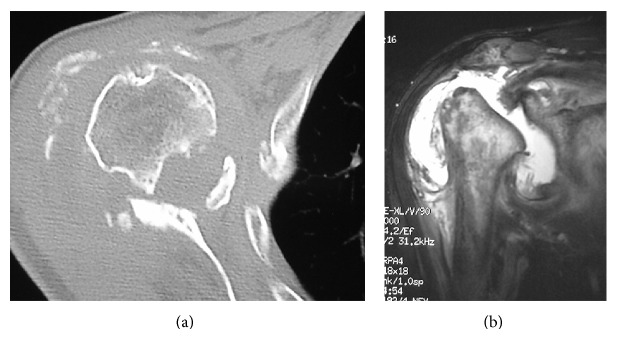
Case  1. Imaging of the right shoulder 4 months after the first visit. (a) An axial computed tomography view demonstrates a destroyed humeral head, displaced bony fragments outside of the greater tuberosity, and destruction of the glenoid. (b) T2-weighted magnetic resonance image (oblique coronal plane) shows the joint destruction, joint effusion, and a rotator cuff tear.
